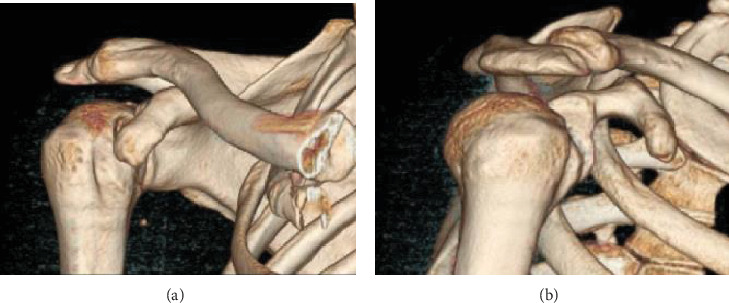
3D CT reconstruction of an AP (a) and lateral view of the shoulder (b).
